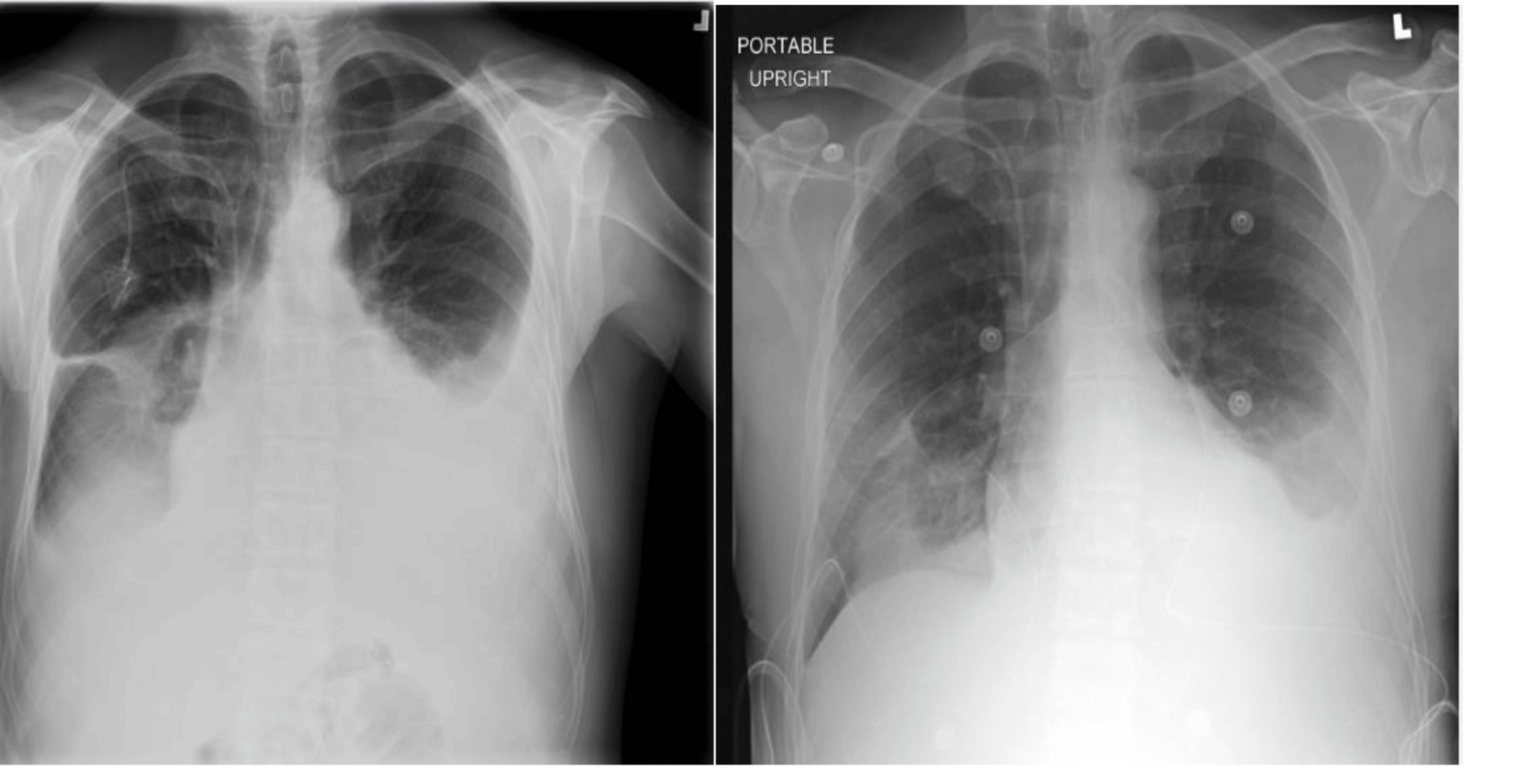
Chest X-ray showing bilateral moderate to large pleural effusions (left) and post indwelling pleural catheter placement with improvement in effusions (right).
